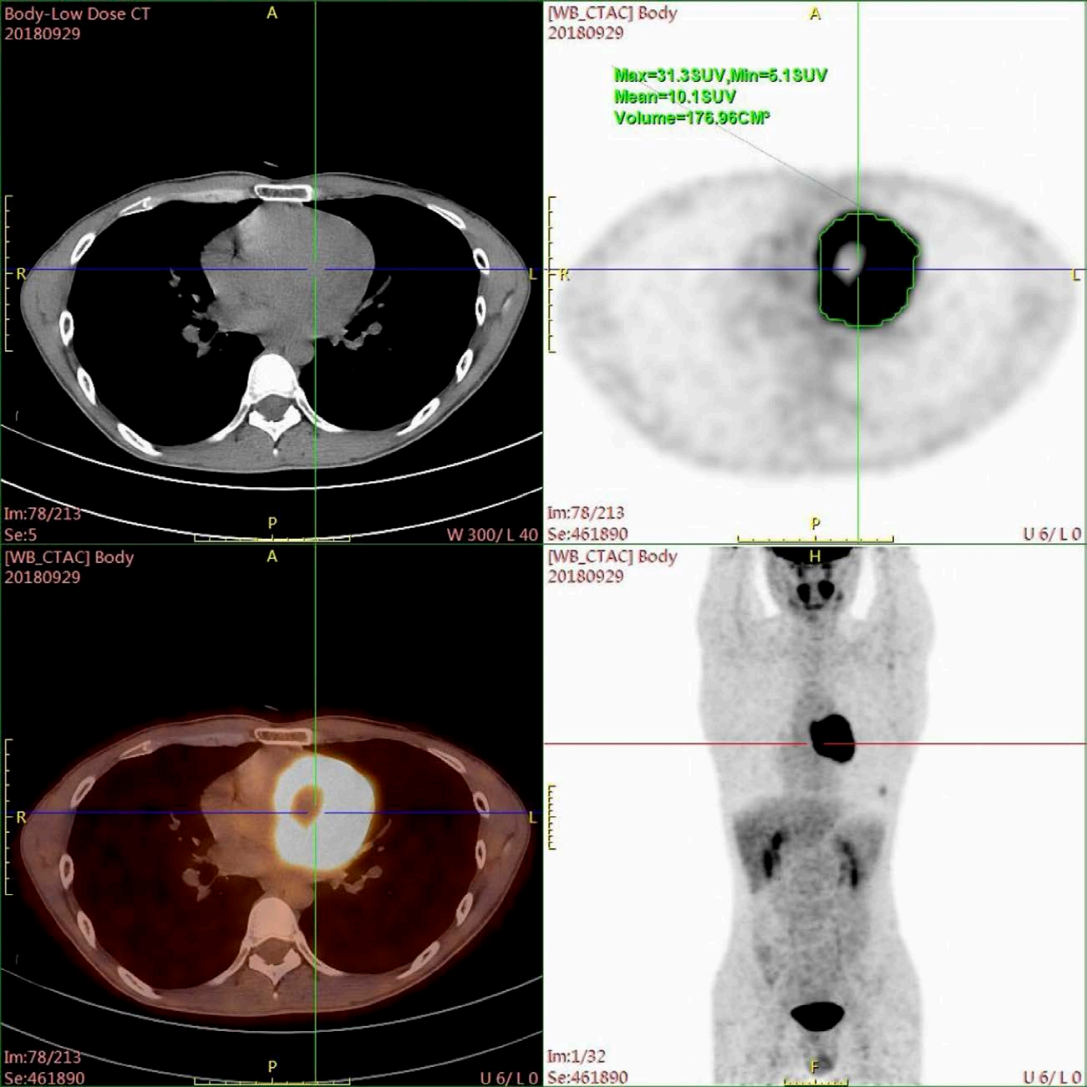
Positron emission tomography-computed tomographic scan demonstrated a marked accumulation of ^18^F- fluorodeoxyglucose in middle mediastinal mass.
